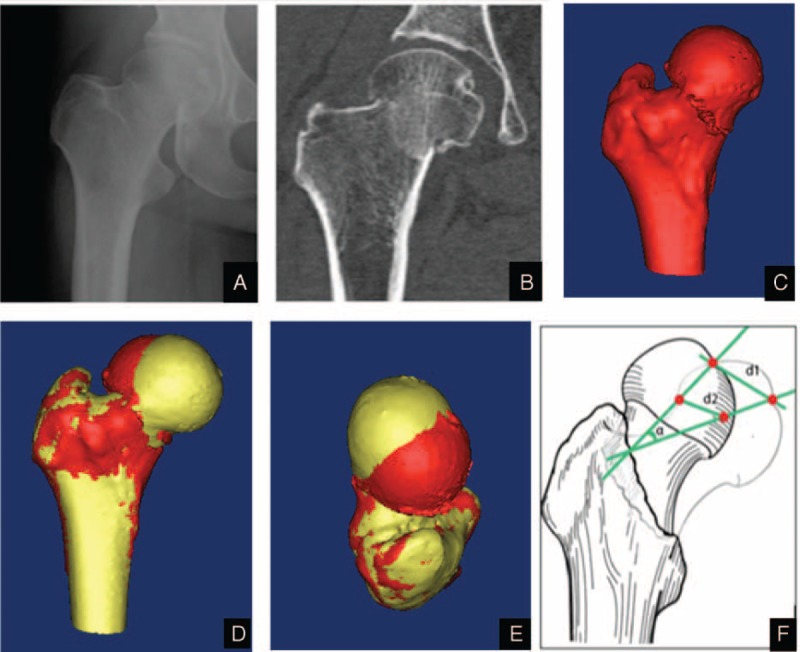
A Garden stage I right femoral neck fracture. (A) Antero-posterior radiograph. (B) Coronal scanning of computed tomography. (C) 3-dimensional model of femoral neck fracture. (D) Front view of bilateral femoral 3-dimensional model. (E) Top views of bilateral femoral 3-dimensional model. The red model represents femoral model with fracture, and the yellow model represents the healthy femoral model. (F) The figures describe the new measuring method for spatial displacements.
